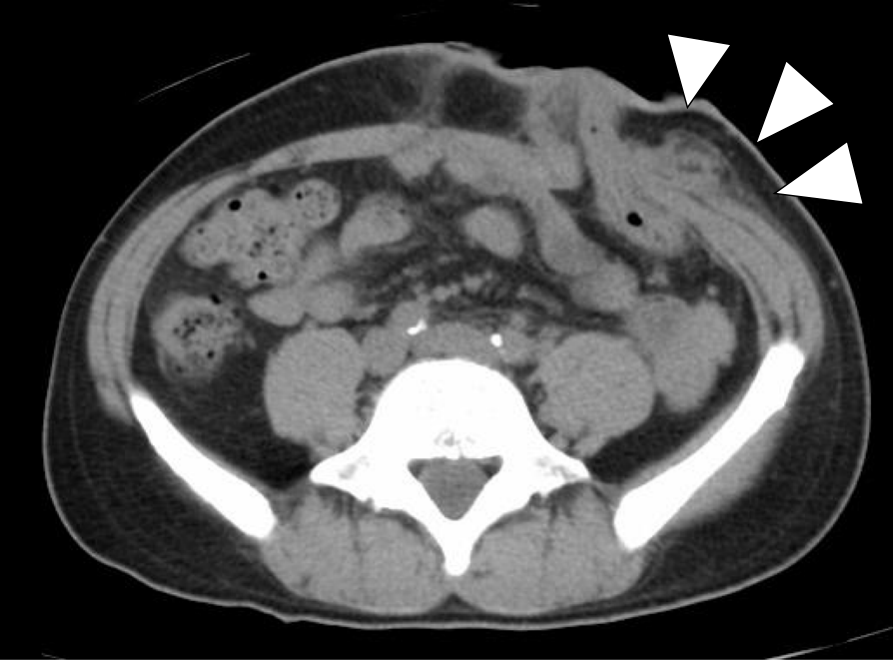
Follow-up computed tomography of the abdomen after the drainage showed abscess decrease (white arrows)
